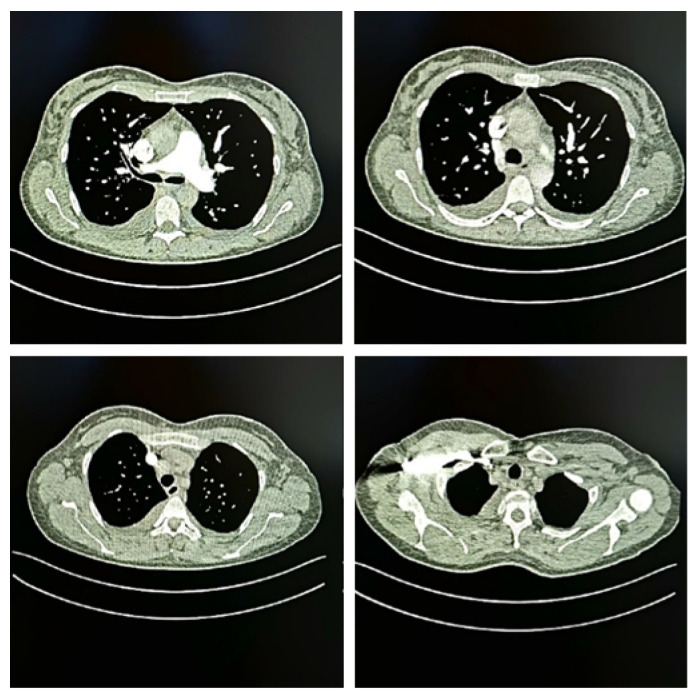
CT pulmonary angiography of the patient presented in this case, showing bilateral pneumonic infiltrates and pleural effusion.
